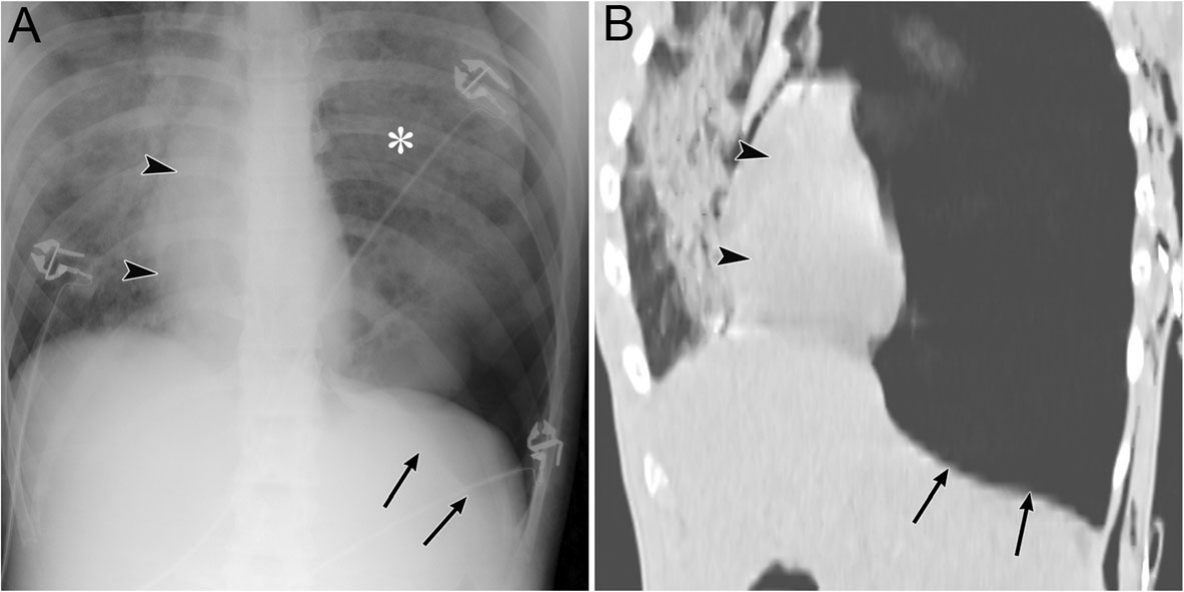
Tension pneumothorax due to traffic trauma in an 18-year-old man. a A supine radiograph shows left pneumothorax with a rightward mediastinal shift (black arrowheads) and partly inverted left hemidiaphragm (black arrows). The left lung is collapsed with a contusion (asterisk). b A coronal CT image clearly depicts a rightward mediastinal shift (black arrowheads) and an inverted left hemidiaphragm (black arrows)
